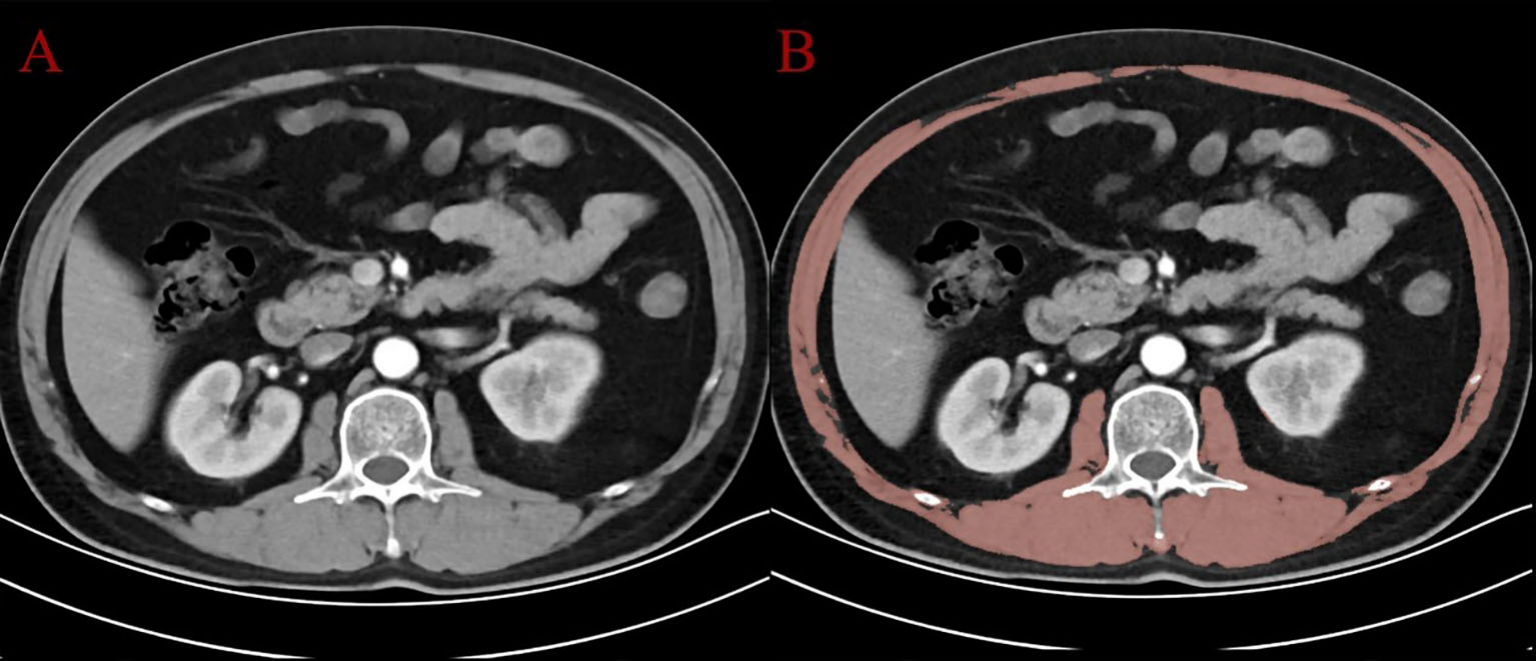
CT image at the L3 level: (A) original axial slice; (B) segmented skeletal muscle (in red) using 3D Slicer.
